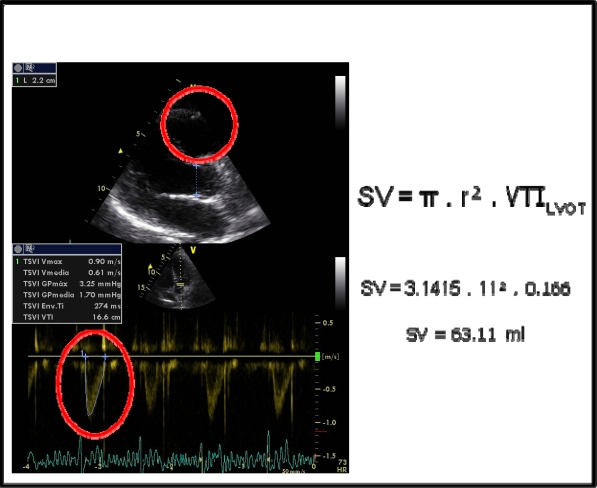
Stroke volume echocardiographic calculus: According to the equation SV= π x r² x VTI_LVOT_ = 59 ml (Normal). (SV= systolic volume, VTI_LVOT_= Left ventricular outflow tract velocity-time integral, r = left ventricular outflow tract ratio).
